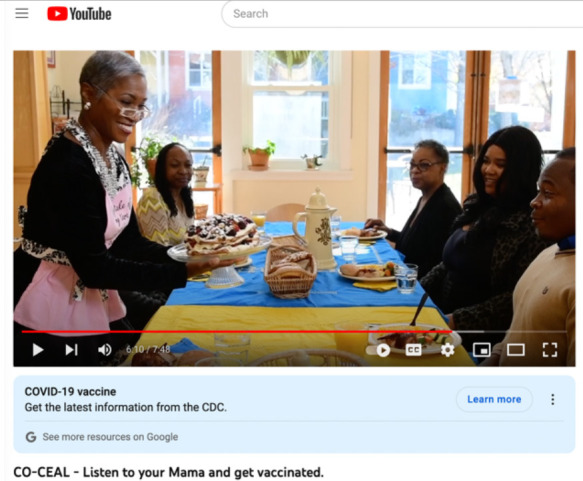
Urban Latino/a/x	•Together we can *(juntos si se puede)* •Vaccine is safe and easy to get •Cuidate tu para cuidarnos a nosotros. Mantenos seguros. Vacunate. #JuntosSiSePuede •Take care of you to take care of us. Keep us safe. Get the vaccine. #JuntosSiSePuede	•Spanish and English language bus ads and bench signs, posters and rack cards •Featured rapid–CT participants •Messages focused on care of/for yourself and others, save lives, hope, work together as a community •Safety and accessibility of vaccine •Vaccination protected community to just individual	•Bus ads and bench signs throughout community •Placed posters and rack cards in community areas, social media •Rack cards and masks distributed at local events
